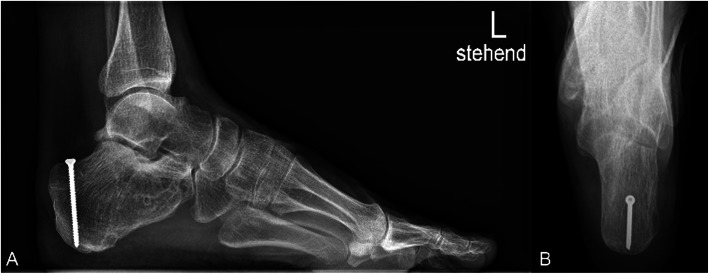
Case 1 Radiographs at follow-up Weightbearing left foot lateral (A) and calcaneus axial (B) 40 months post-surgery, AOFAS score 95
